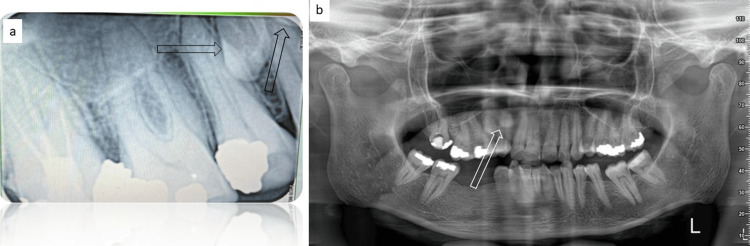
Conventional radiograph (IOPAR and OPG) views (a) Intraoral periapical radiograph (IOPAR) faintly showing the presence of a supernumerary tooth in relation to right maxillary premolars (black arrows). (b) Orthopantomogram (OPG) showing the presence of a supernumerary tooth with two crowns (white arrow).
